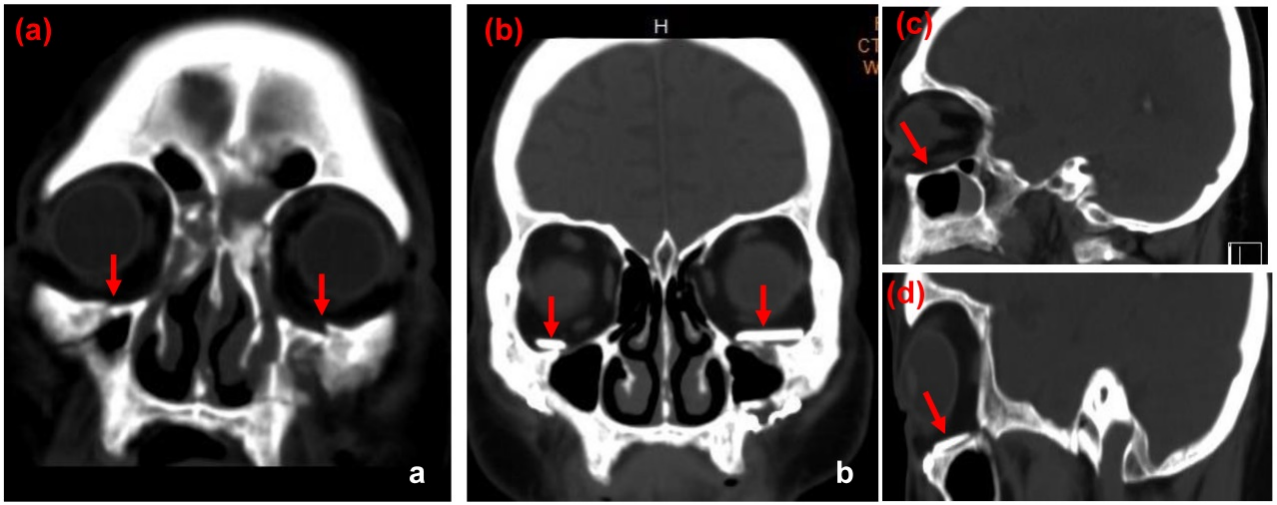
CT images of case 2. The comparison between preoperative CT scan of impaired orbital wall and postoperative CT scan of the orbital wall with ABCcolla® Collagen Bone graft (red arrows). (a) Pre-operative CT image with the coronal view. Fractures of bilateral inferior orbital walls can be seen (red arrows). (b) Post-operative CT image with the coronal view. ABCcolla® Collagen Bone grafts were implanted into bilateral inferior orbital walls (red arrows). (c) Post-operative CT image with the sagittal view. Fractures of bilateral inferior orbital walls are shown (red arrows). (d) Post-operative CT image with the sagittal view. ABCcolla® Collagen Bone graft was implanted into bilateral inferior orbital walls (red arrows).
